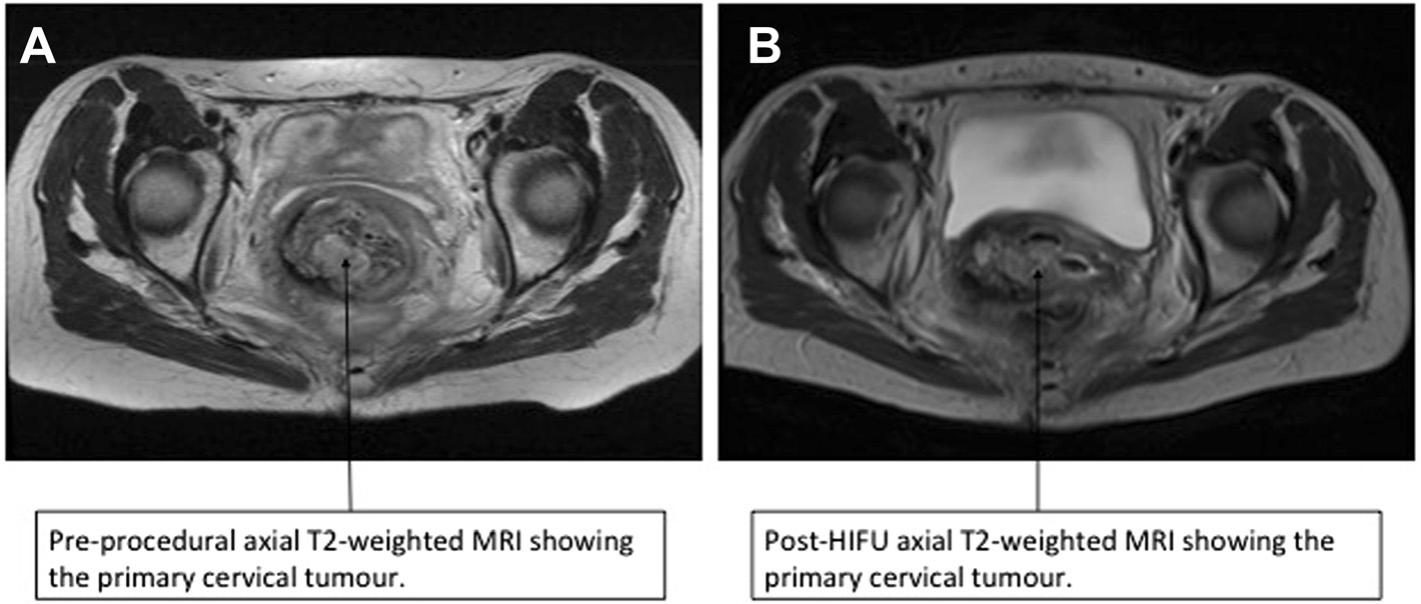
Pre- and post-treatment MRI changes. Axial T2-weighted MRI at the level of the pelvic side wall showing the primary cervical tumour at baseline (a) and post-treatment (b). A significant tumour burden remains, although, the AP length of the lesion has reduced in size from 4.5 to 3.4 cm
